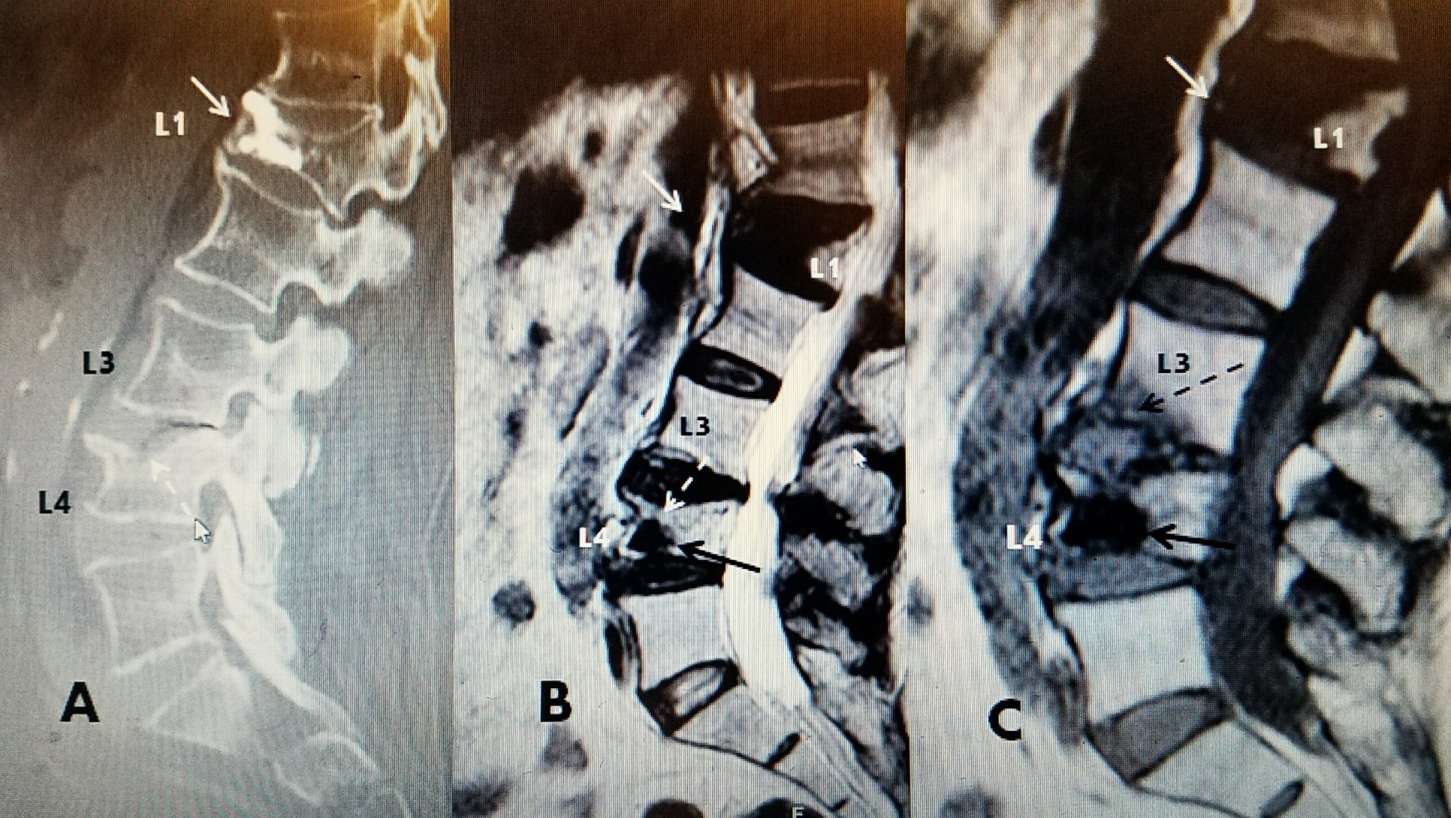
85-year-old male with an L4 compression fracture of superior endplate and kyphoplasty inferior to the fracture A: Computerized tomography scan (CT) showing L4 with a 20% anterior-superior endplate fracture (dotted back arrow). There was a previous vertebroplasty anteriorly at L1 (solid white arrow). B; Magnetic resonance image (MRI) T2 image post-kyphoplasty with polymethylmethacrylate (PMMA) showing cement anteriorly and inferiorly at L4 (solid black arrow), but below the fracture of the superior endplate (dotted white arrow). C: Sagittal MRI T1 one month after B showing new edematous change in the adjacent vertebrae in the anterior inferior part of L3 (dotted black arrow). The cement from the kyphoplasty is below the L4 superior endplate fracture (black arrow). The old L1 kyphoplasty cement is unchanged (solid white arrow).
